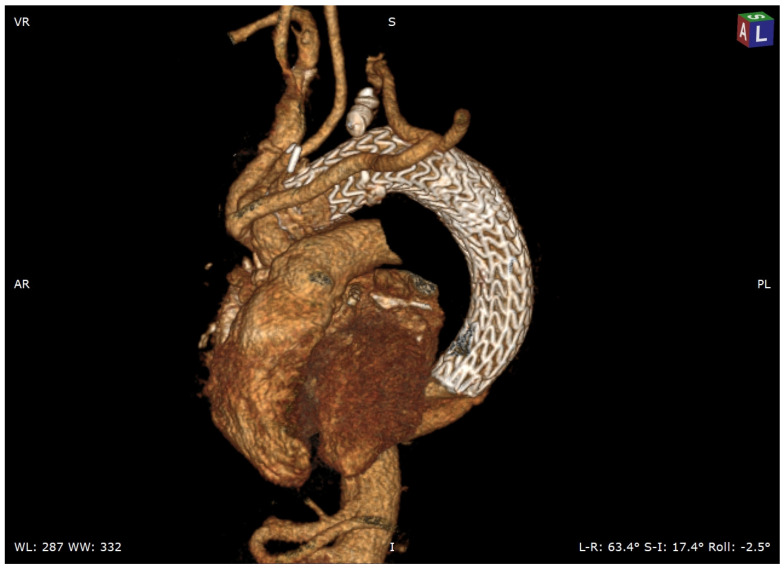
Three-dimensional CT scan: final result after endovascular distal extension and left subclavian artery origin plug occlusion.
